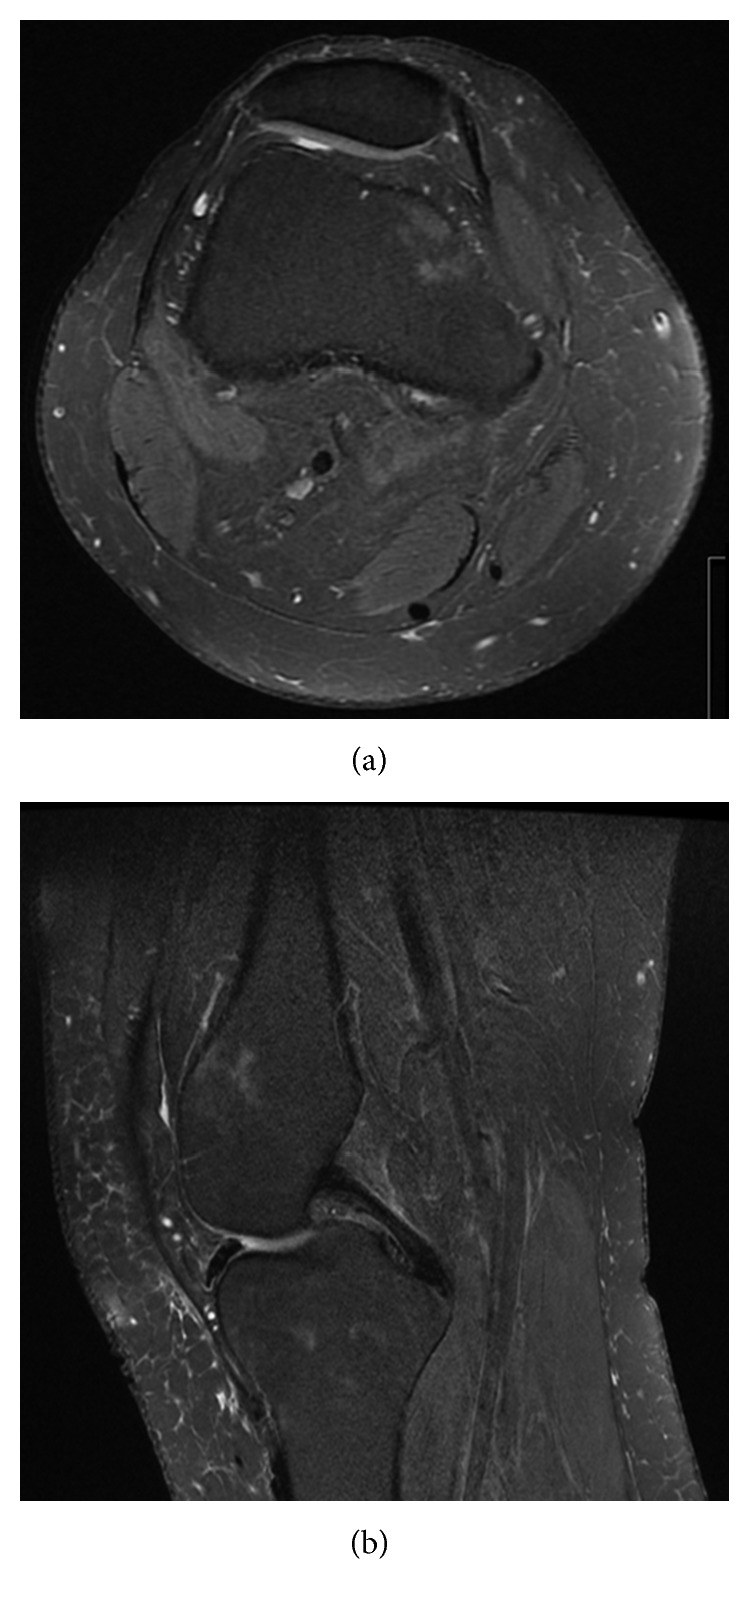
MRI (T2 Fat Sat; (a) axial and (b) sagittal images of left knee) showing medial femoral condyle marrow oedema.
